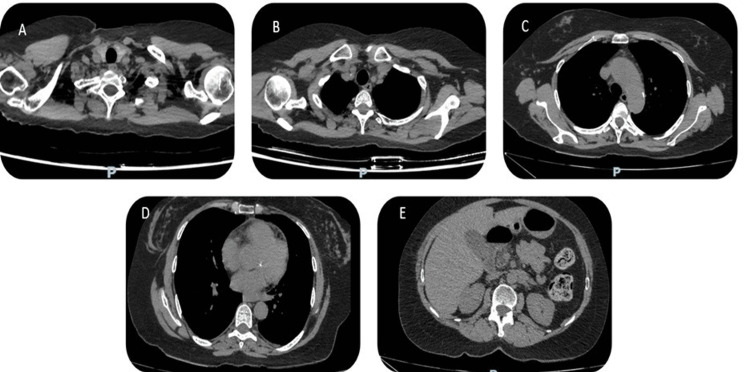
Chest CT scan Image A (upper section): visualization of the right subclavian artery showing signs of stenosis in its distal portion, just at the exit of the quadrilateral space. Image B (middle section): shows aneurysmal dilation of the ascending aorta with a diameter of 44 mm. The aortic walls exhibit regular circumferential thickening greater than 3 mm, indicative of aortitis. Image C (lower section): Thickening and enhancement of the descending aortic wall, suggesting large-vessel vasculitis, compatible with giant cell arteritis. Image D (thoracic view): Evidence of thoracic aorta dilation with involvement of the aortic arch. No signs of thrombosis. Image E (Abdominal section): Evaluation of the abdominal system, with no evidence of aneurysmal dilation or significant stenosis in the main abdominal vessels.
